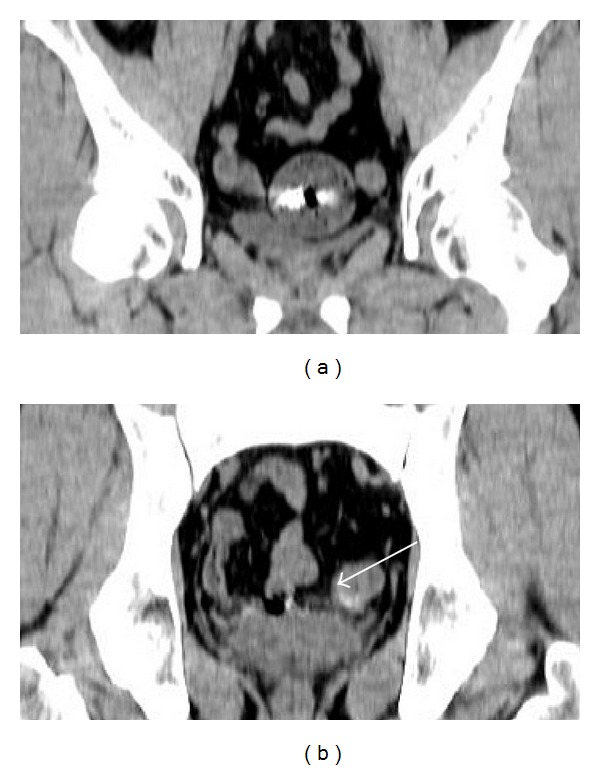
Coronal view by CT showed CE capsule retention in the small intestinal lumen with dilatation and fluid collection (a), and the finding was suspicious for stenosis ((b), arrow).
